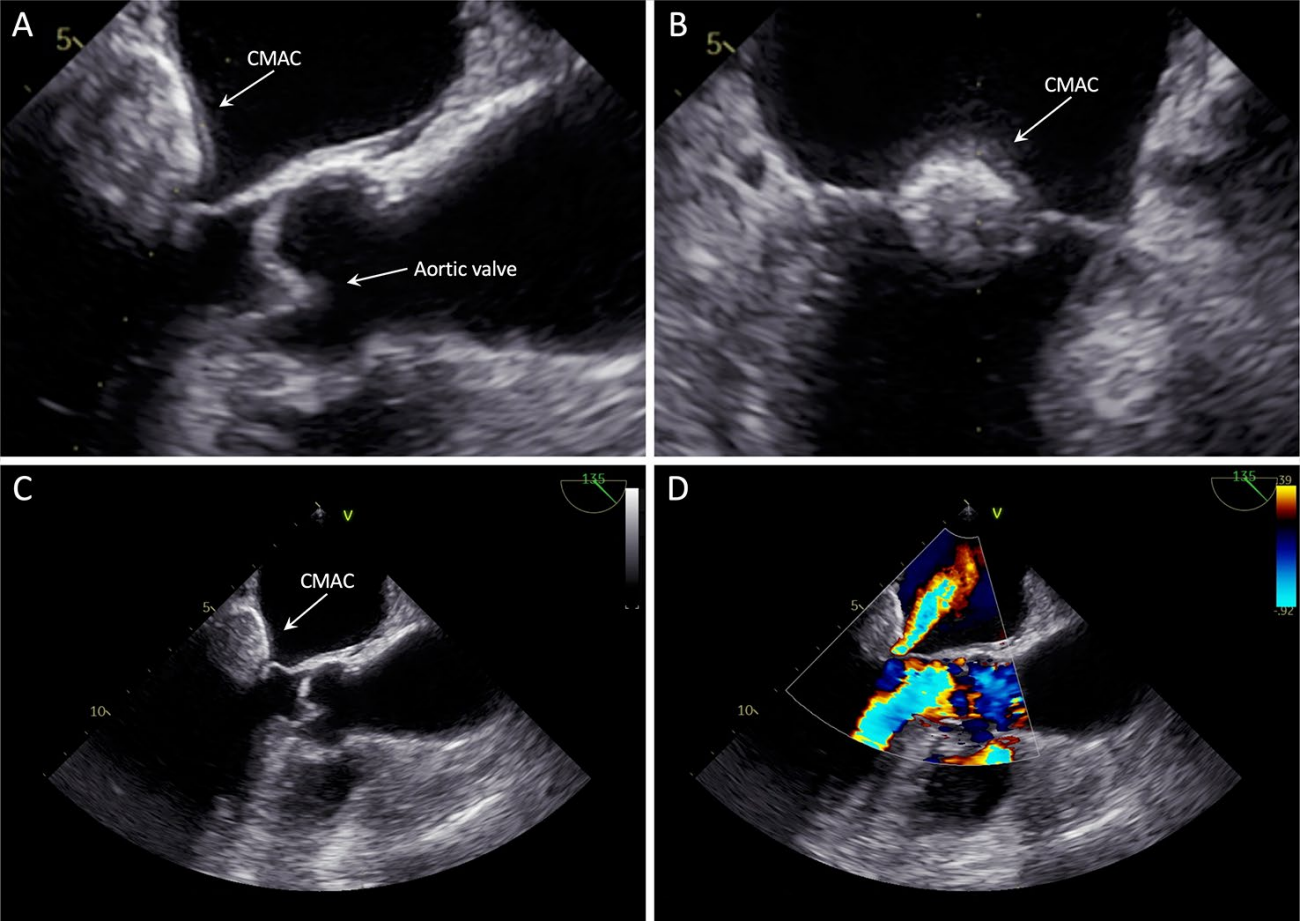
Transesophageal echocardiography showing a well-defined, smooth-bordered echo-dense mass with central echolucency at the posterior peri-annular region of the mitral valve causing a mild mitral regurgitation. *CMAC* caseous mitral annular calcification
